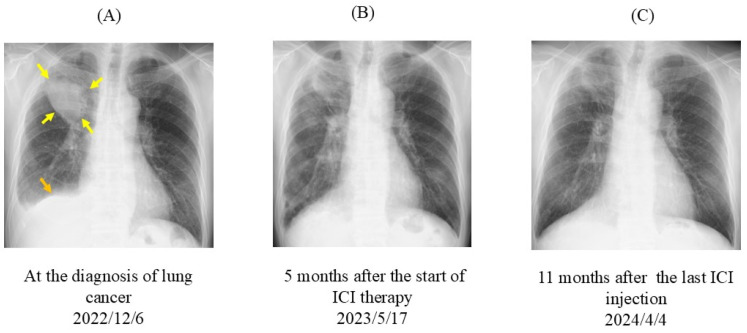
Chest radiographs. (A) At lung cancer diagnosis, a large mass (yellow arrows) and pleural effusion (orange arrow) were observed in the right lung. (B) Five months after the start of chemotherapy and ICI therapy, the tumor size had reduced. (C) Eleven months after the last ICI injection, tumor expansion was not observed. ICI, immune checkpoint inhibitor.
